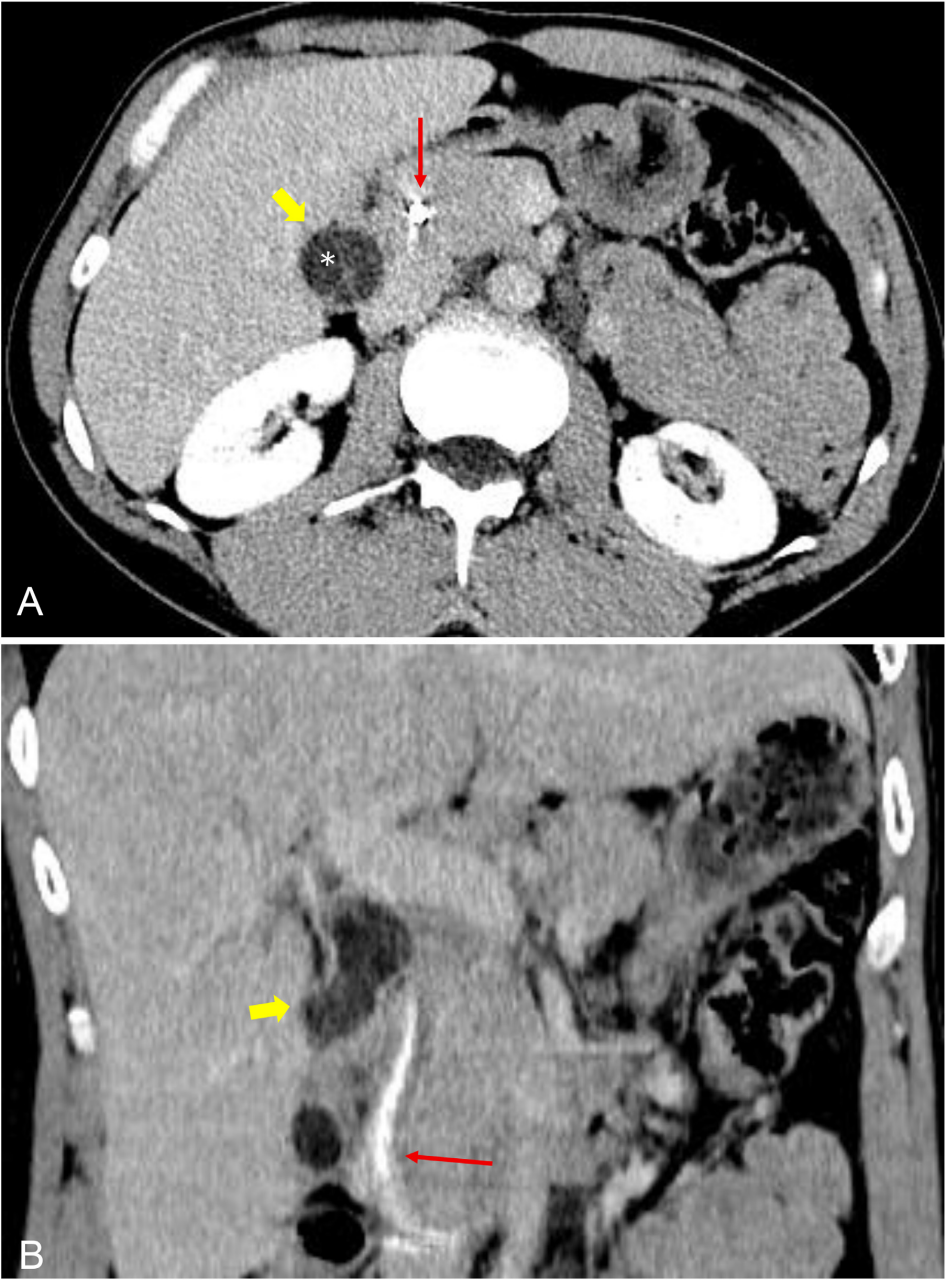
CT of the abdomen, contrast enhanced, portal venous phase: (A) Transverse CT image demonstrates the gallbladder (yellow arrow) with internal septations (asterisk) and adjacent biliary stent (red arrow). No mural nodularity, soft tissue attenuation mass, or internal calcification is demonstrated. (B) Coronal CT image demonstrates a multiseptated lesion within the gallbladder (yellow arrow) and a biliary stent (red arrow).
